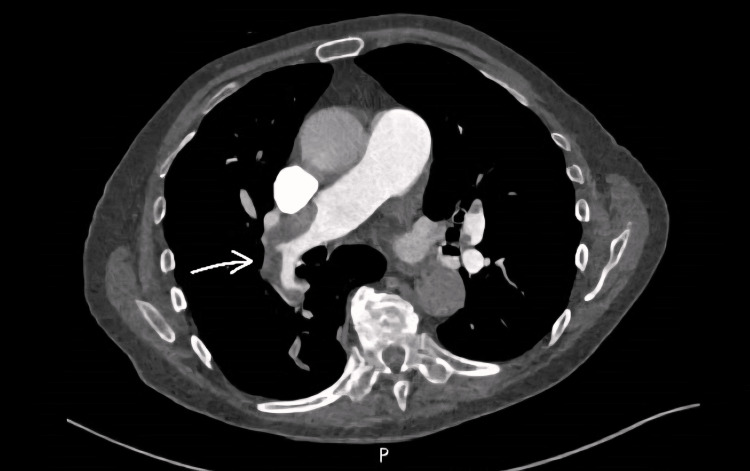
Computed tomography pulmonary angiogram (CTPA) Pulmonary embolism noted involving the right main pulmonary artery (arrow), the right interlobar artery, and multiple segmental arteries on both sides. Diagnosis of massive pulmonary embolism was based on imaging evidence.
